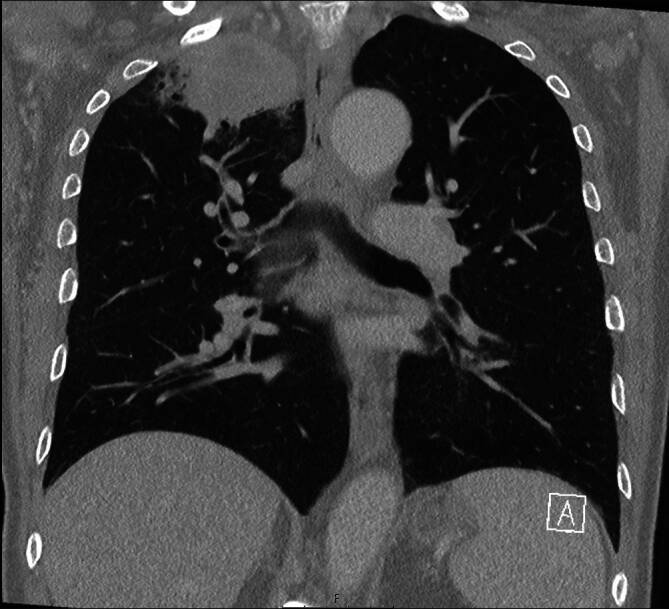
Frontale CT Thorax mit apikaler pulmonaler Raumforderung
